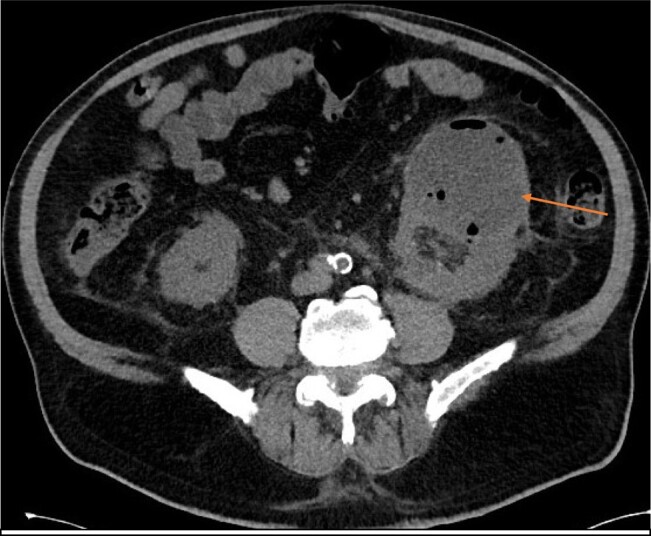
The noncontrast image of the computed tomography abdomen showing a left renal abscess with fluid and air (marked with an arrow).
